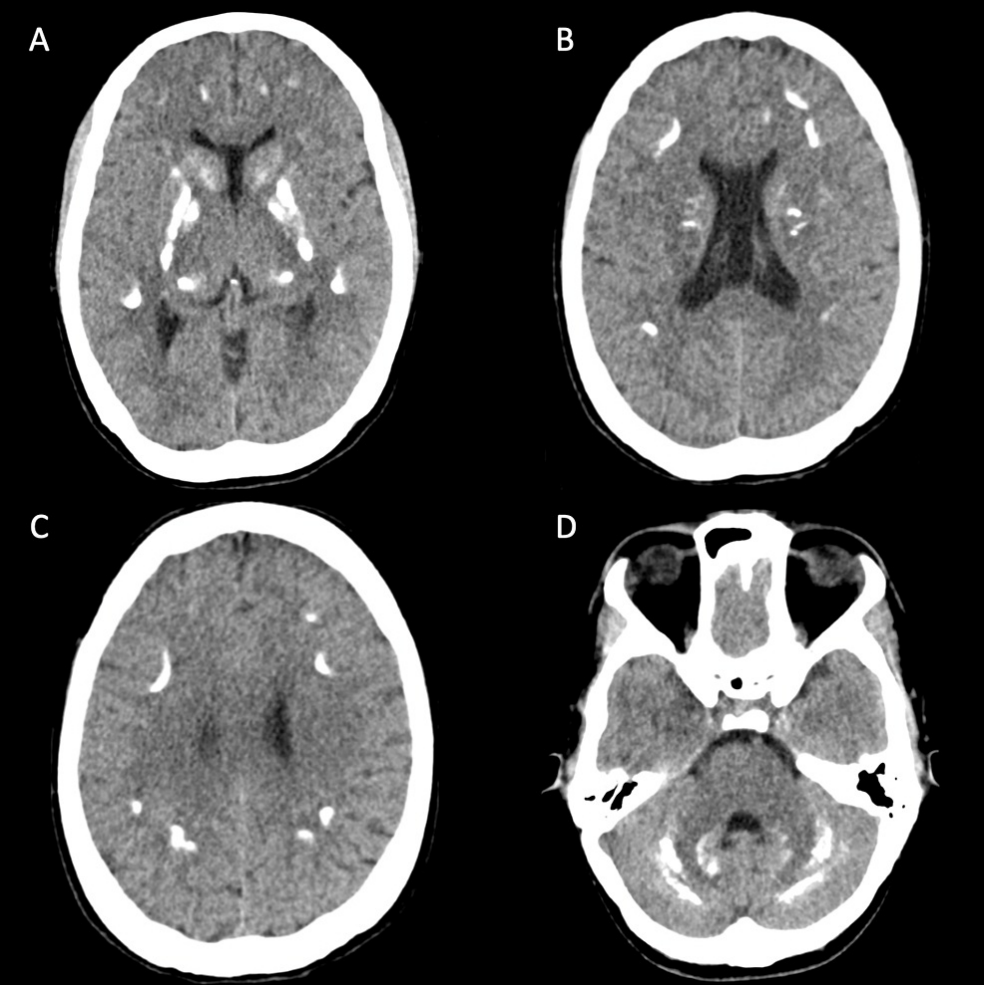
Brain CT without contrast (A) Axial nonenhanced head CT without contrast demonstrates fairly symmetric dense calcifications in the caudate, globus pallidi, putamen, and the thalami. (B, C) Images obtained at a higher level show multiple foci of dense calcifications in the centrum semiovale and at the gray-white matter junction. (D) Extensive diffuse hyperdense calcifications are also present throughout the cerebellum.
